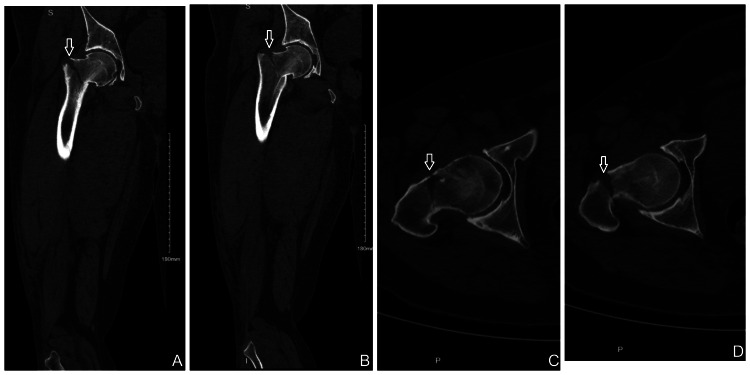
Coronal (A) and (B) and axial (C) and (D) computed tomography images of the right hip demonstrating a basicervical fracture of the femoral neck (white arrows).
